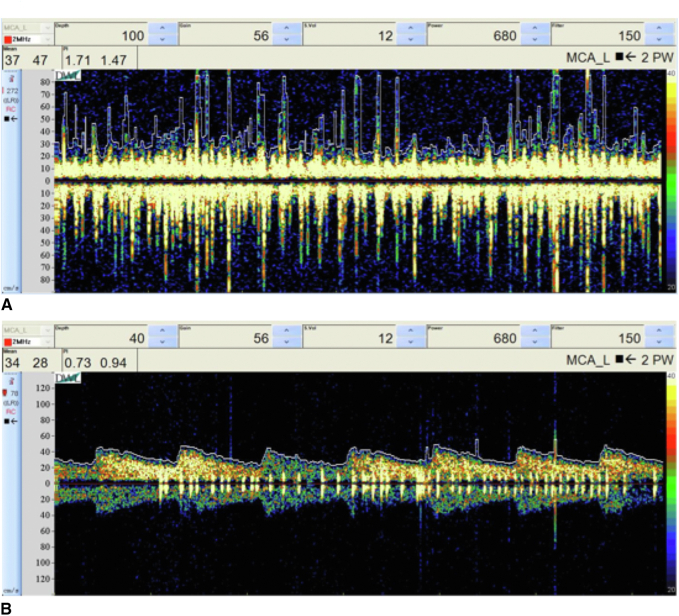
Examples of transcranial Doppler (*TCD*) waveforms exhibiting high microembolic signals in the left middle cerebral artery in patients receiving (A) venoarterial (*VA*) and (B) venovenous (*VV*) extracorporeal membrane oxygenation (*ECMO*). *MCA*, Middle cerebral artery.
